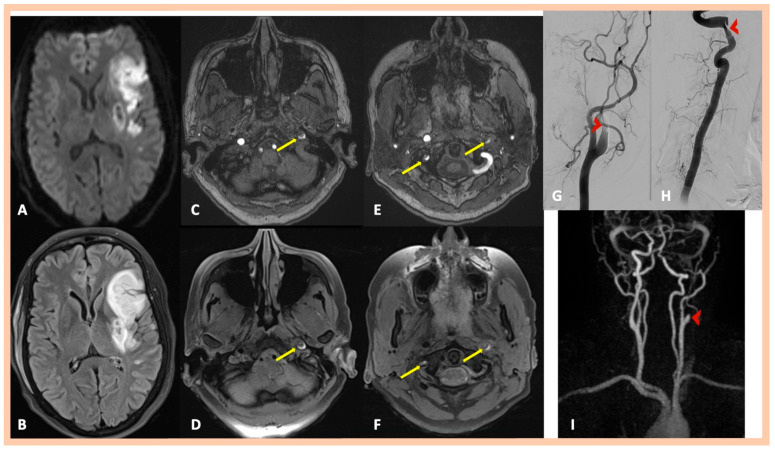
Neuroimaging findings—Patient #2. High-resolution 3 Tesla brain MRI showing a left middle cerebral artery infarction (Panel (A): diffusion restriction in axial DWI sequence; Panel (B): hyperintense signal in axial FLAIR imaging). Double lumen and intraluminal hematoma along the left ICA are depicted in Time-of-Flight (TOF) MRA (Panel (C)) and 3D fat-saturated black-blood T1-weighted high-resolution (3 Tesla) vessel wall imaging (Panel (D)), respectively. An asymptomatic spontaneous dissection with double lumen and intramural hematoma along the V3 segment of the right vertebral artery was also detected using the same MR sequences (Panel (E): TOF MRA—Panel (F): 3D fat-saturated black-blood T1 SPACE). Digital subtraction angiography confirmed the left ICA dissection, revealing a “flame-shaped” occlusion (Panel (G)) and also revealed focal stenosis (approximately 80%) along the V3 segment of the right vertebral artery, indicative of intramural hematoma (Panel (H)). MRA showing the left ICA occlusion as well (Panel (I)).
